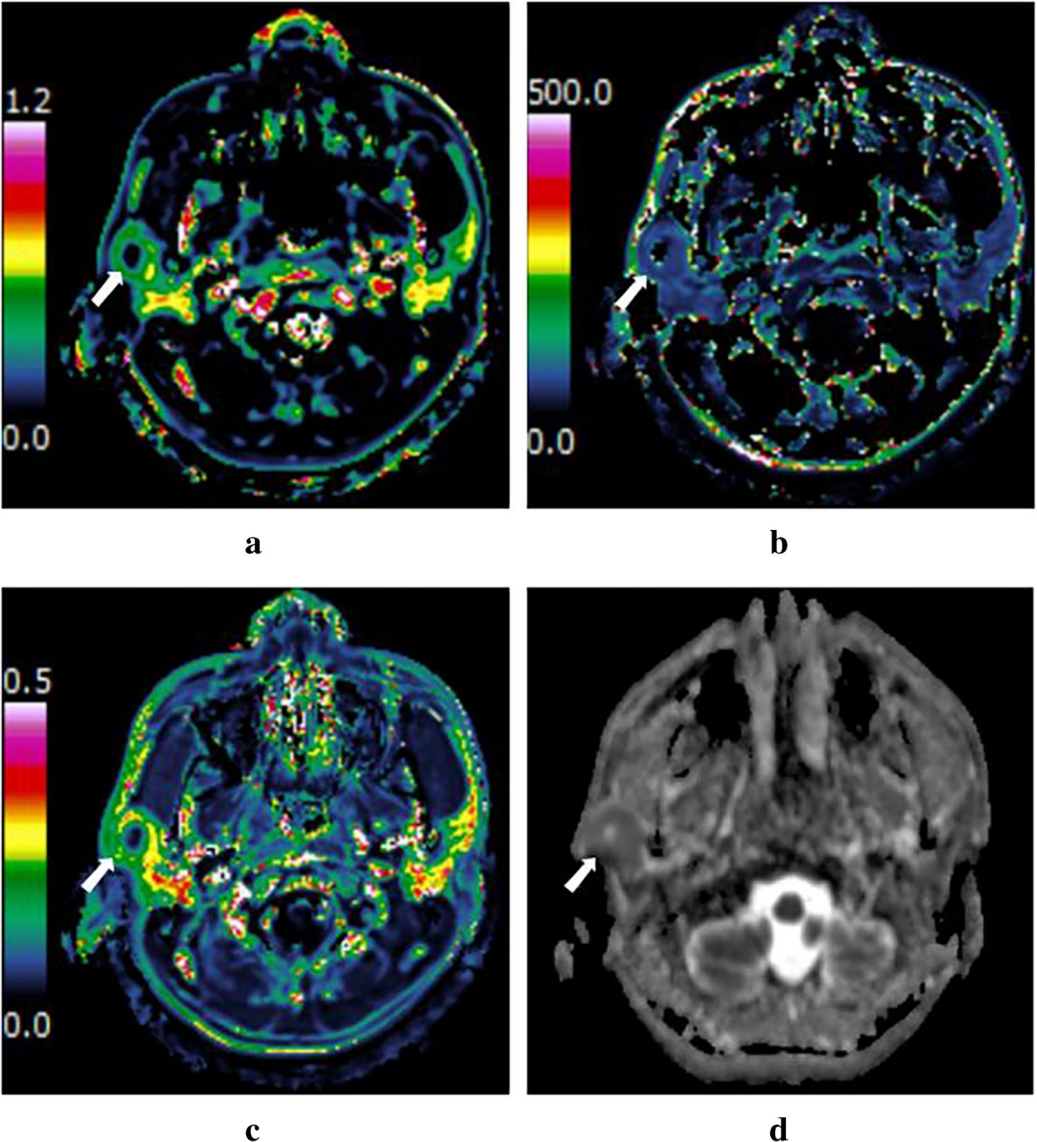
A 24-year-old man with mucoepidermoid carcinoma in the right parotid gland (white arrow). DCE-MRI parameter maps showed the *K*_ep_ (a), *V*_e_ (b), and *K*^trans^ (c) values of 0.90 min^−1^, 0.61, and 0.55 min^−1^, respectively. d
